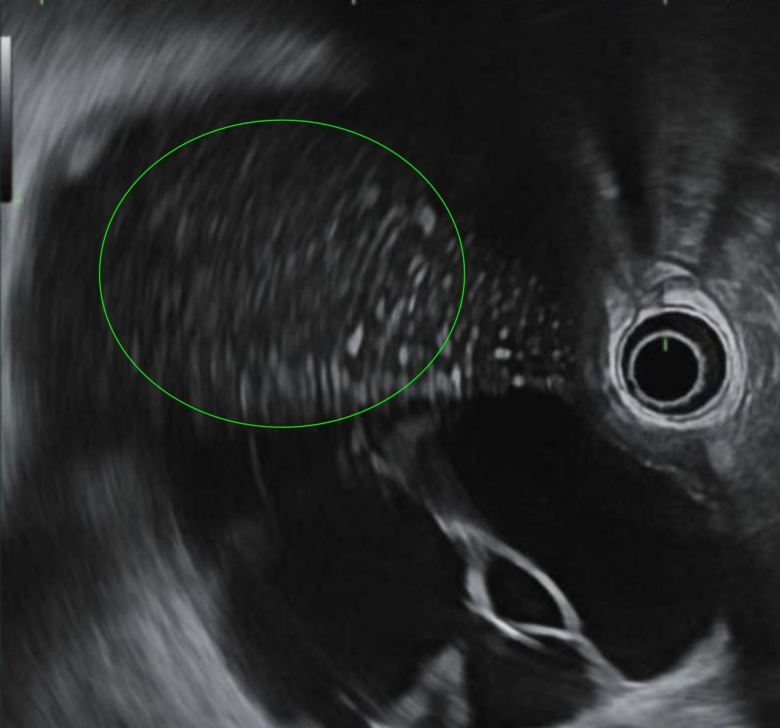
Endoscopic ultrasound of pancreatic cystic mass: EUS showing a 12 cm complex cystic mass encompassing the distal pancreas. EUS = endoscopic ultrasound.
